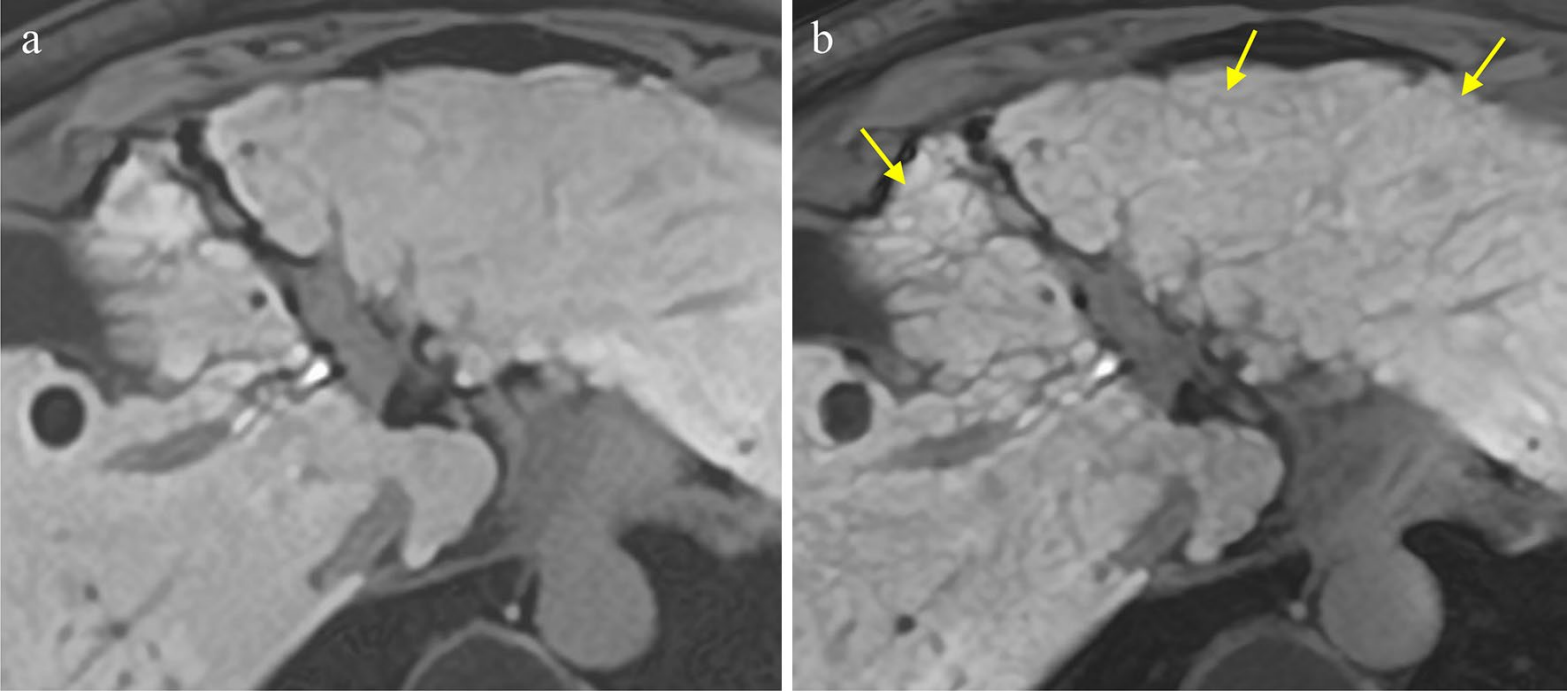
MR images in a 74-year-old woman with liver cirrhosis. a HBP image with CS using AiCE. b High-precision HBP image with modified Fast 3D mode wheel and PIQE. Hepatic regenerative nodules with hypointense thin septa (arrows) were more clearly visualized in high-precision HBP image with modified Fast 3D mode wheel and PIQE (b), compared with HBP image with CS using AiCE (a)
